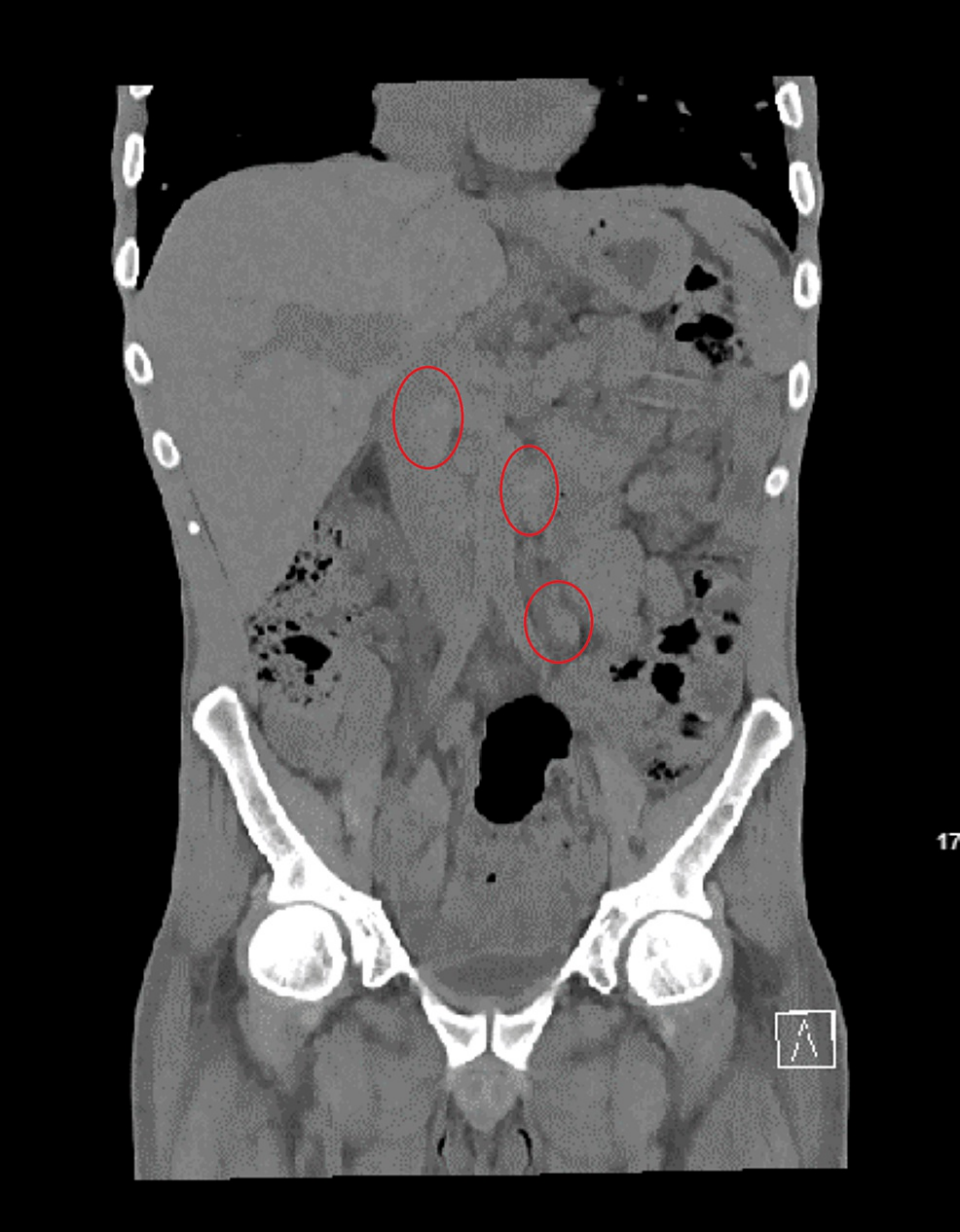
Computed tomography of the abdomen and pelvis without contrast coronal view (lesions in the circle).
